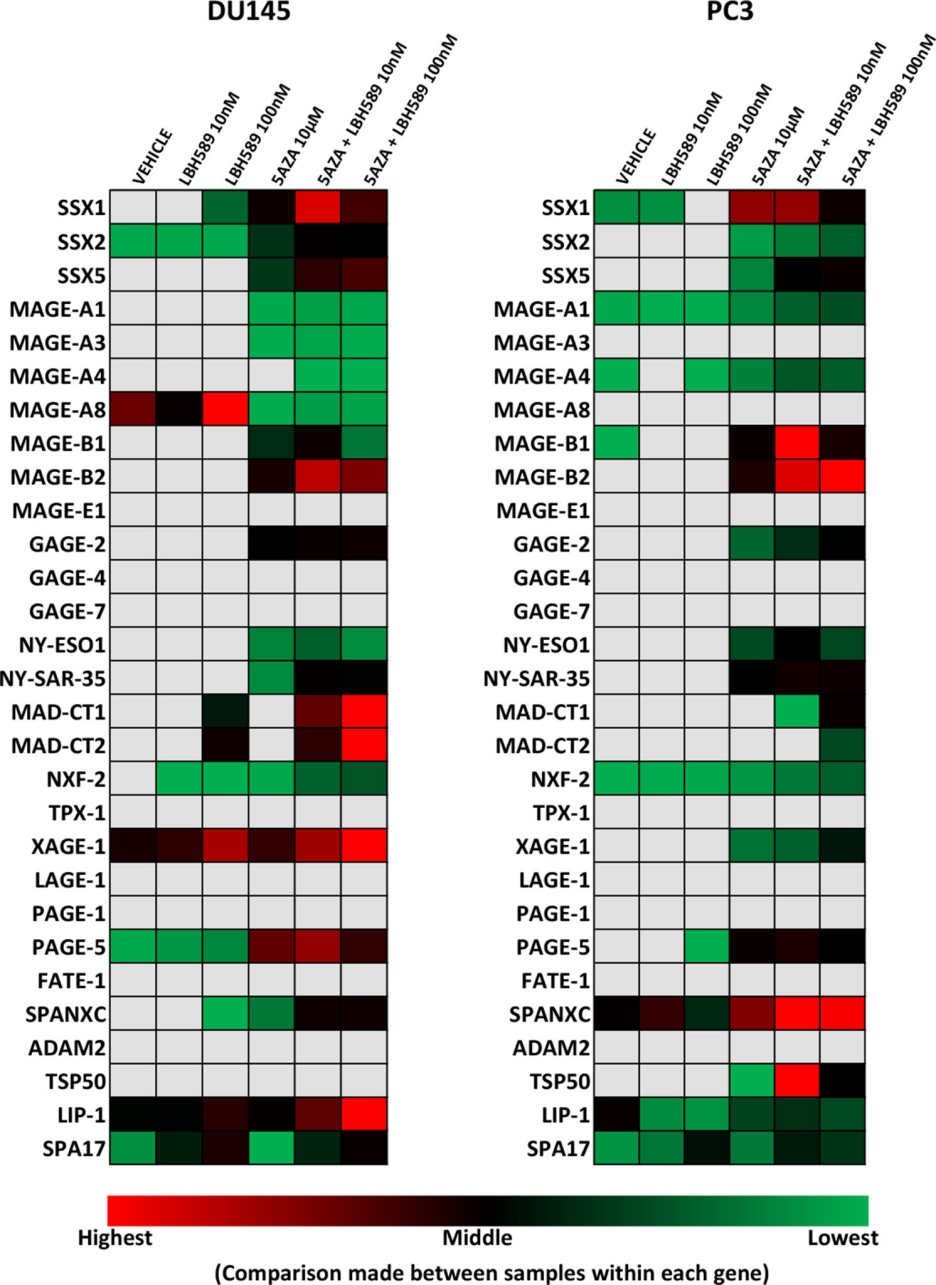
Quantitative analysis of relative expression of CTA mRNA in androgen-independent PC cell lines treated with EMAs RNA was evaluated by qRT-PCR for expression relative to an internal control transcript (P0) following EMA treatment of DU-145 and PC3 cells. qRT-PCR was performed using primers specific for each gene, conducted in triplicate, and repeated in an independent experiment. Expression relative to P0 was color scaled from red (highest) to black (middle; 50th percentile) to green (lowest) for each CTA separately, across all cell lines, thus similar colors between different rows cannot be directly compared. Grey indicates that no relevant expression was detected.
